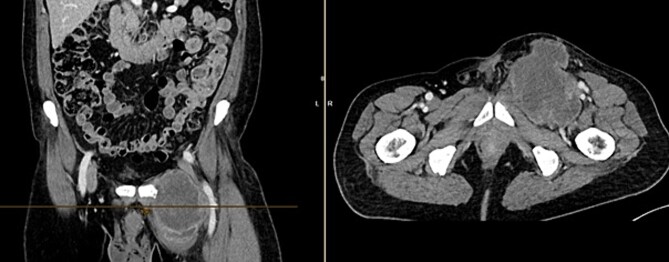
CT of the abdomen from 26 July 2023: size-progressive metastasis in the left groin with infiltration of the adductor muscles; no dynamics in the primarily osteoplastic diffuse osseous metastasis.
